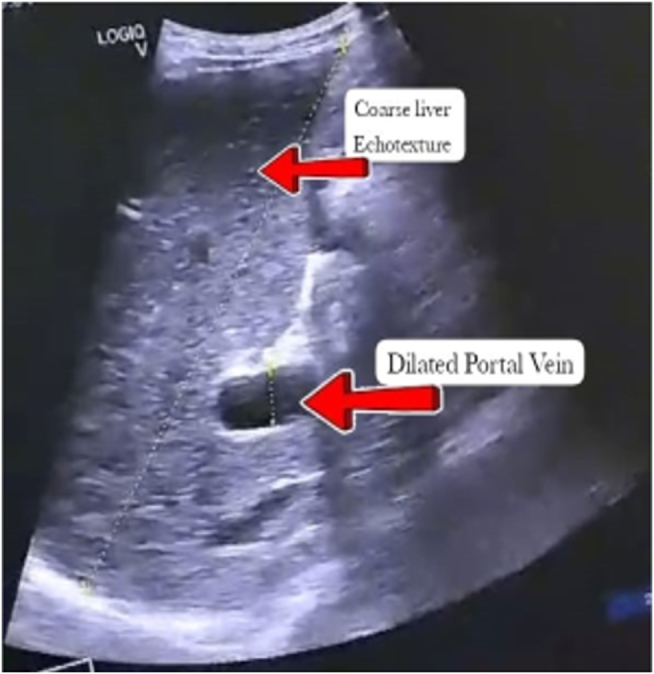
Ultrasonography showing coarse liver texture and a dilated portal vein.
